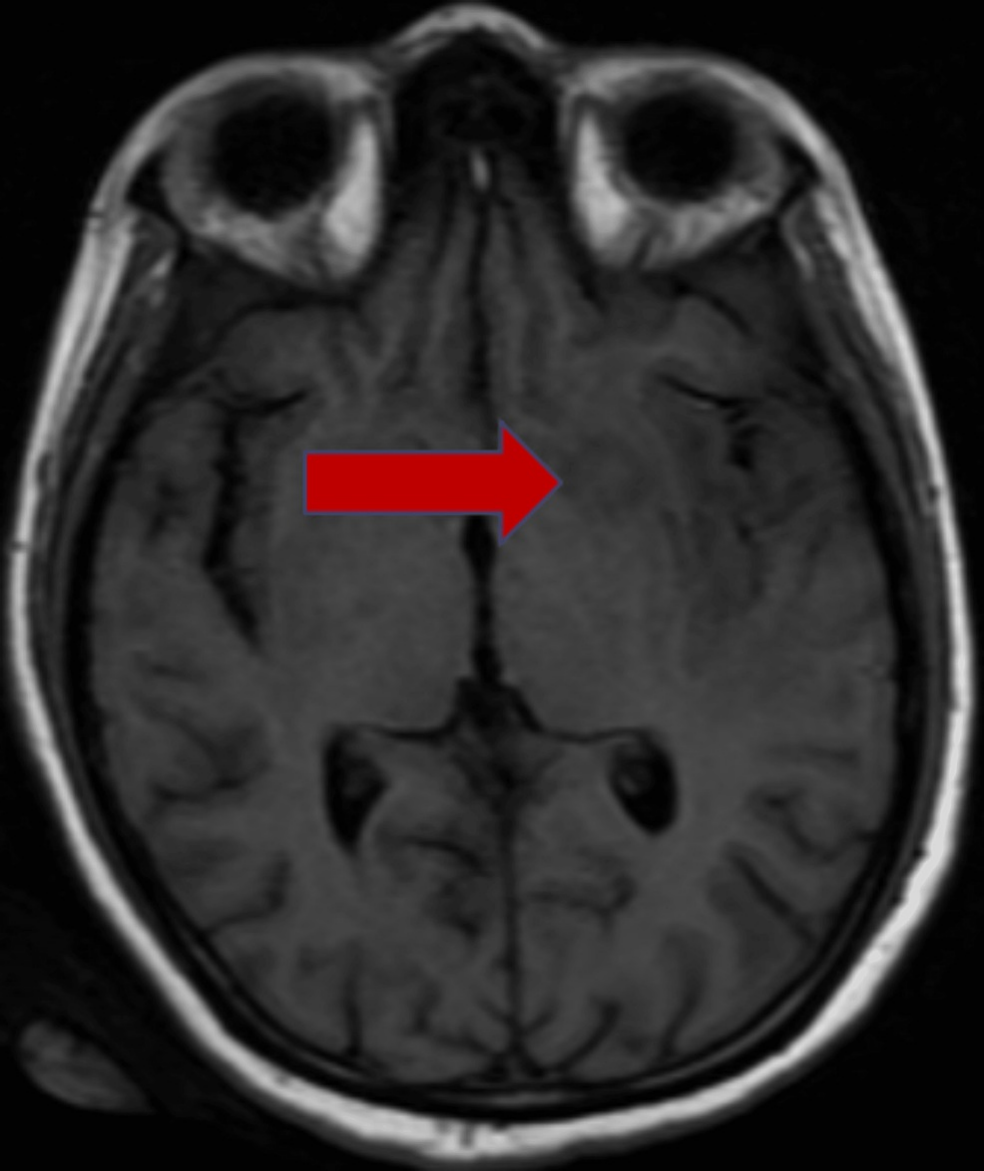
T1-weighted MRI of the brain in axial projection The red arrow marks an isointense ring lesion in the left capsuloganglionic region.
